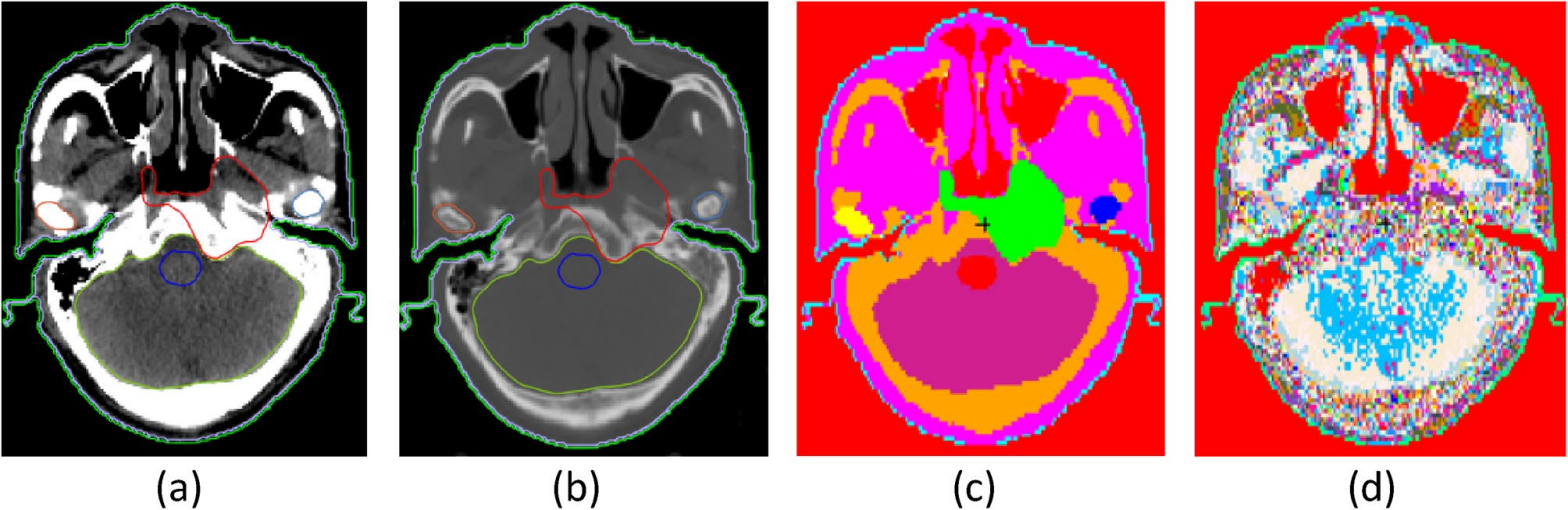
HNC case: (a) CT image in the brain window. (b) CT image in the bone window. (c) Conversion model with specific material from the ICRU-46 report in ROIs. (d) Conversion model with multiple materials that were automatically converted to the elemental compositions and densities by the HU values.
